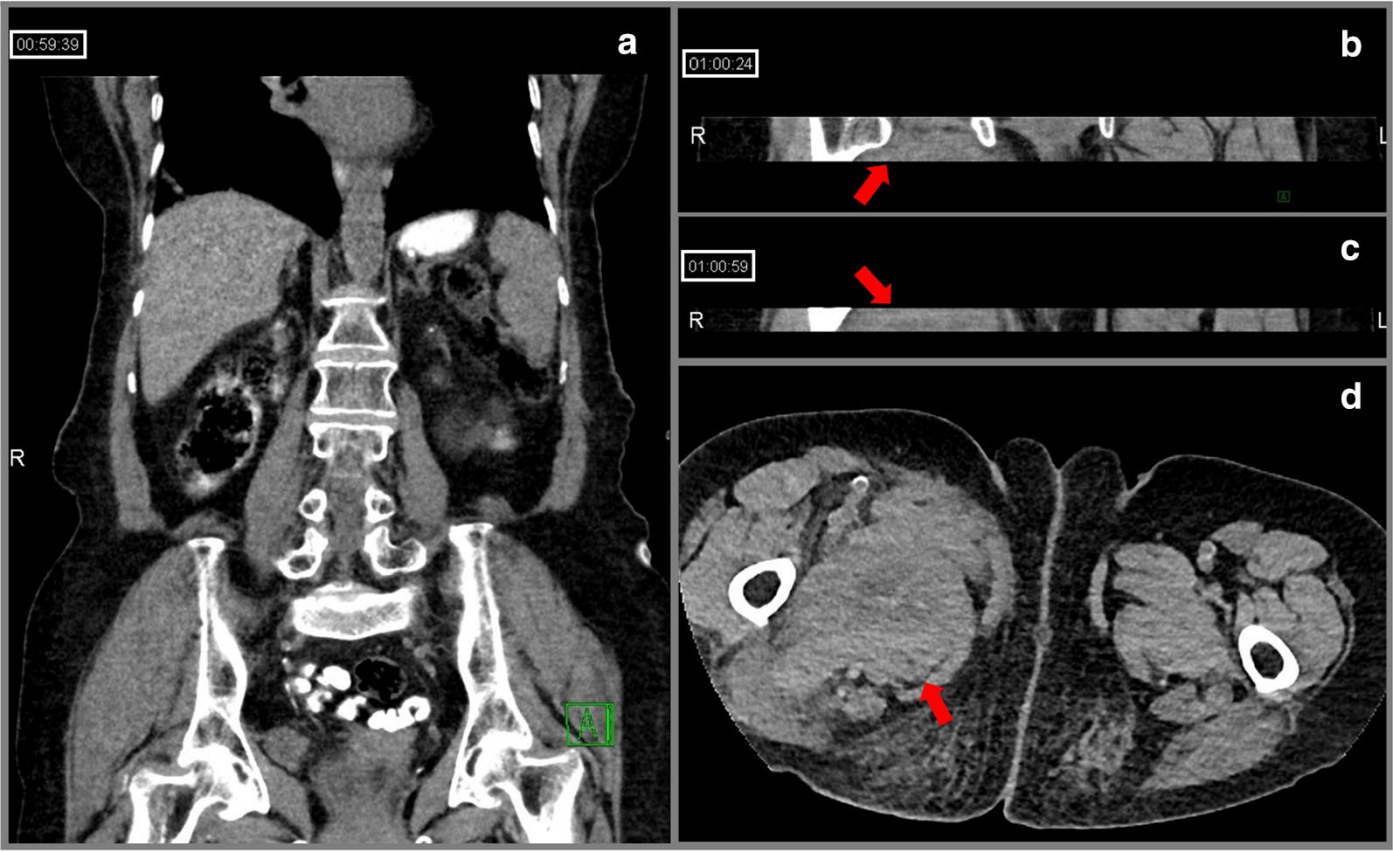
Technique-related (Type 6) error with poor communication. An 88-year-old female patient using an injectable anticoagulant was admitted to the emergency room with right lower quadrant pain. No finding to explain the symptom was observed on abdominal CT (a). Right inguinal swelling developed during follow-up and inguinal US revealed a large hematoma in this region. When the abdominal CT was evaluated retrospectively, it was noticed that, apart from the sections examined by the on-duty radiologists, two more series consisting of only 10–15 axial slices showing inguinal regions were separately uploaded to the PACS together with their coronal reformats (b, c). It was later learned that the CT technician had difficulty adjusting the field of view and had to take a few extra slices to cover the level of the symphysis pubis. However, he did not inform the on-call radiologists about this situation. Since the images were sent to the PACS piece by piece due to a technical error, and the radiologists were not informed about the problem due to lack of communication, the diagnosis of a clearly seen right inguinal hematoma was delayed (red arrows, b, c, d)
